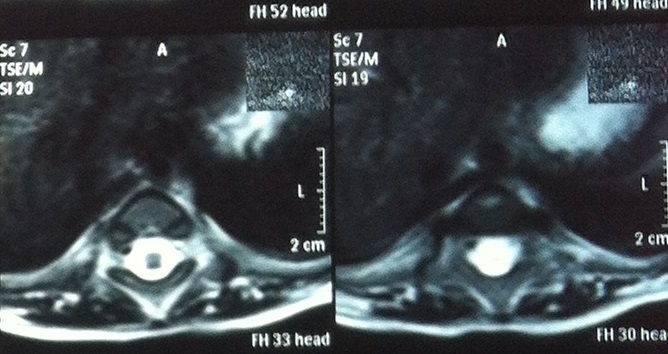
Spinal cord was observed to be terminated at T6 vertebral corpus level, lower than T10 vertebra level, thoracic and lumbar vertebrae and sacrum couldn't be observed according to lumbar MRI
